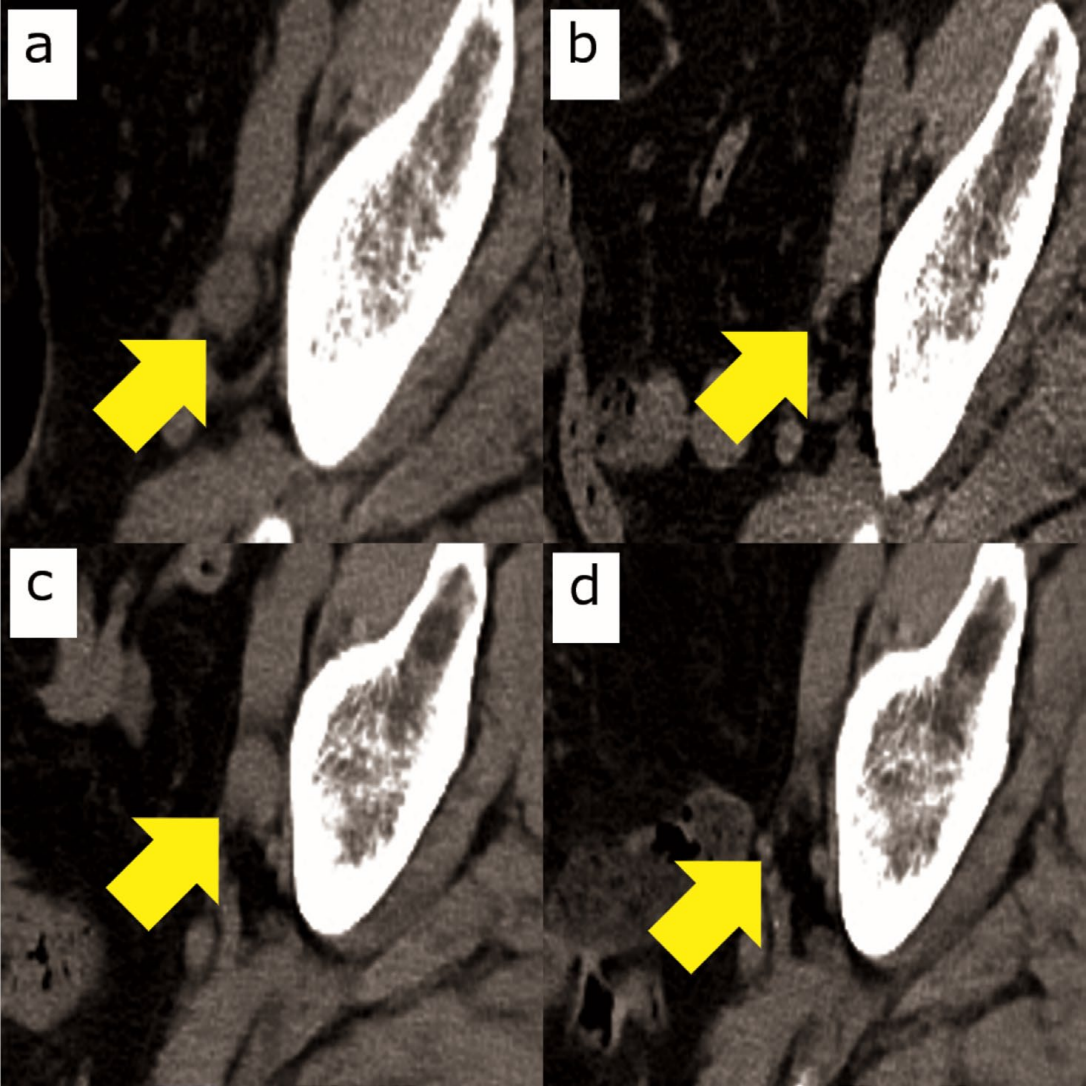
Radiologic course demonstrating recurrence, progression on avelumab maintenance, and complete response to pembrolizumab. Sequential computed tomography (CT) images of the same left obturator lymph node show: all images acquired at comparable axial level and window settings. (a) Recurrent enlargement before gemcitabine–cisplatin chemotherapy (short‐axis 15 mm). (b) Complete response after chemotherapy (< 10 mm). (c) Regrowth after 16 cycles of avelumab maintenance indicating progression (short‐axis 12 mm). (d) Disappearance of the lesion after four cycles of pembrolizumab, confirming a second complete response (< 10 mm).
